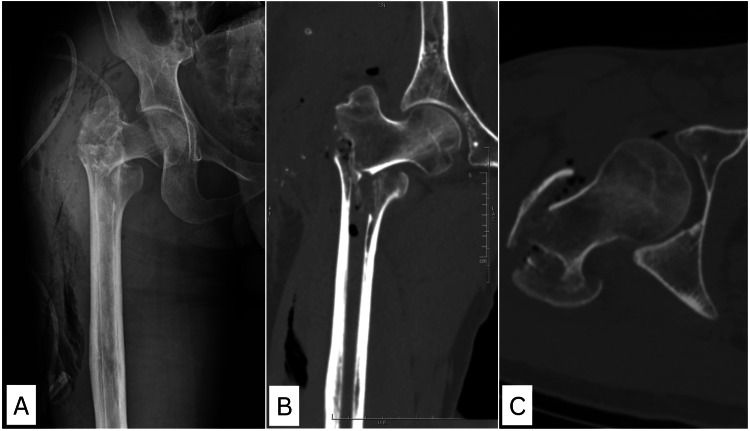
Immediate post-implant removal X-ray image (A) and CT images (B, C) of the right hip showing unstable comminuted trochanteric fracture of the femur
